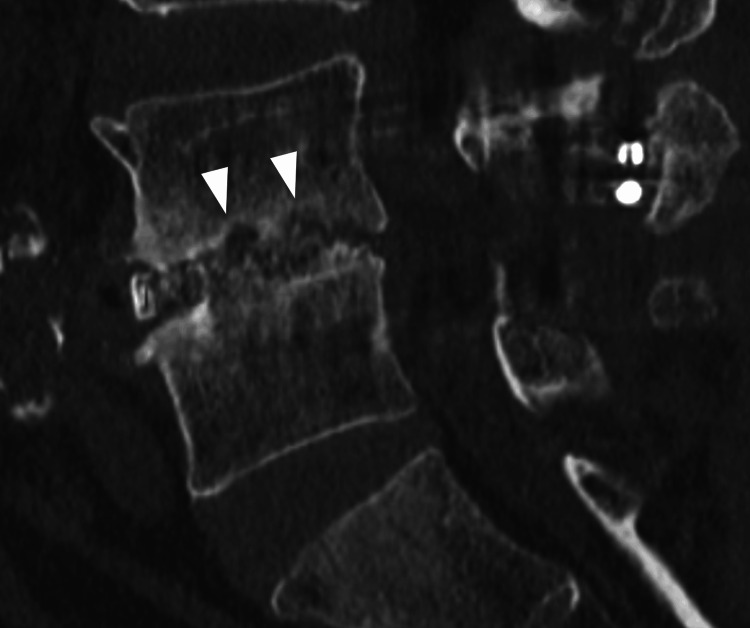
Vertebral endplate cyst sign on CT (arrow head).
